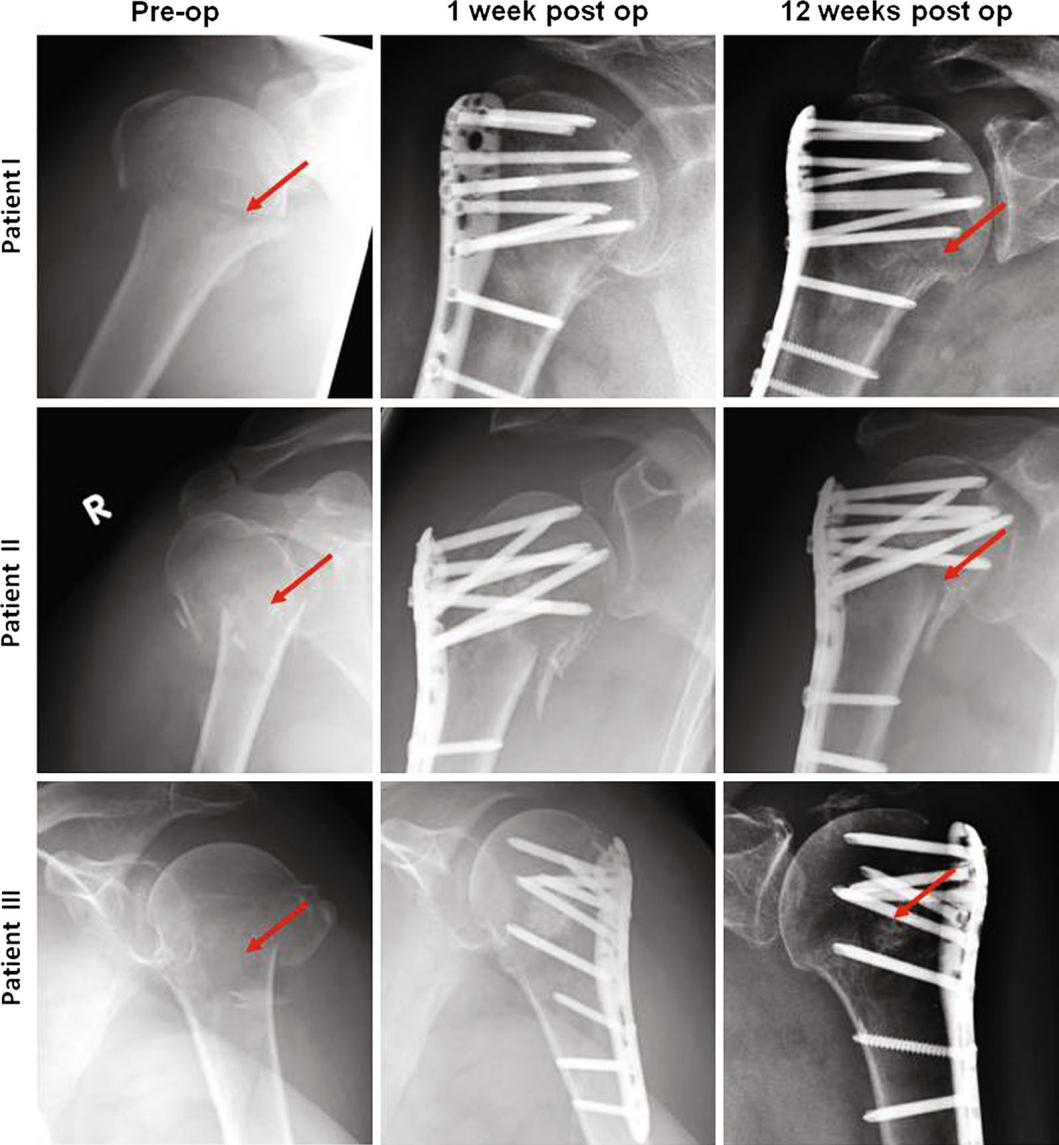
Radiological controls (X-ray) of three representative patients preoperatively, 1–12 weeks after surgery. *Red arrows* show sites of fracture during observation time
